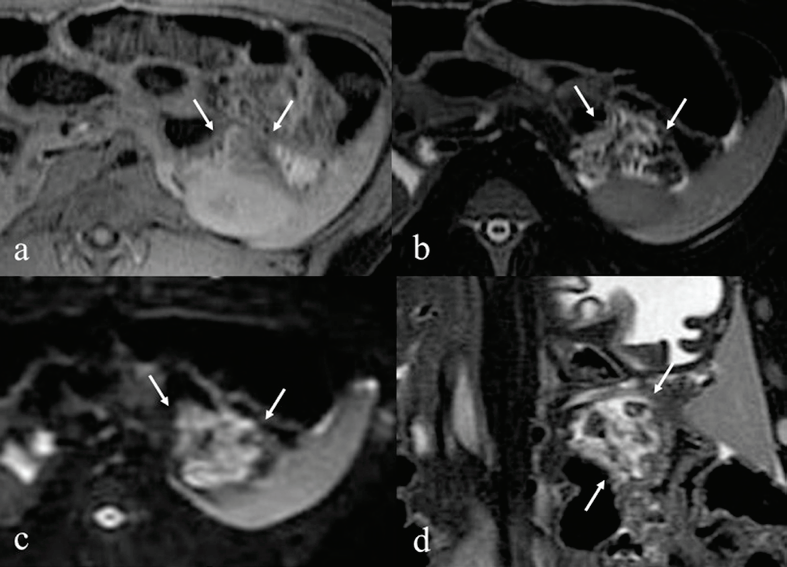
MRI immediately after injection with 2.0 mL ethanol. a: T1-weighted image shows small high and low signal areas measuring 20 x 15 mm in the tail of pancreas. b: T2-weighted image showed linear high signal areas, measuring 42 x 38 mm. c: DWI image showed rounded speckles high signal areas. d: T2 coronal image.
